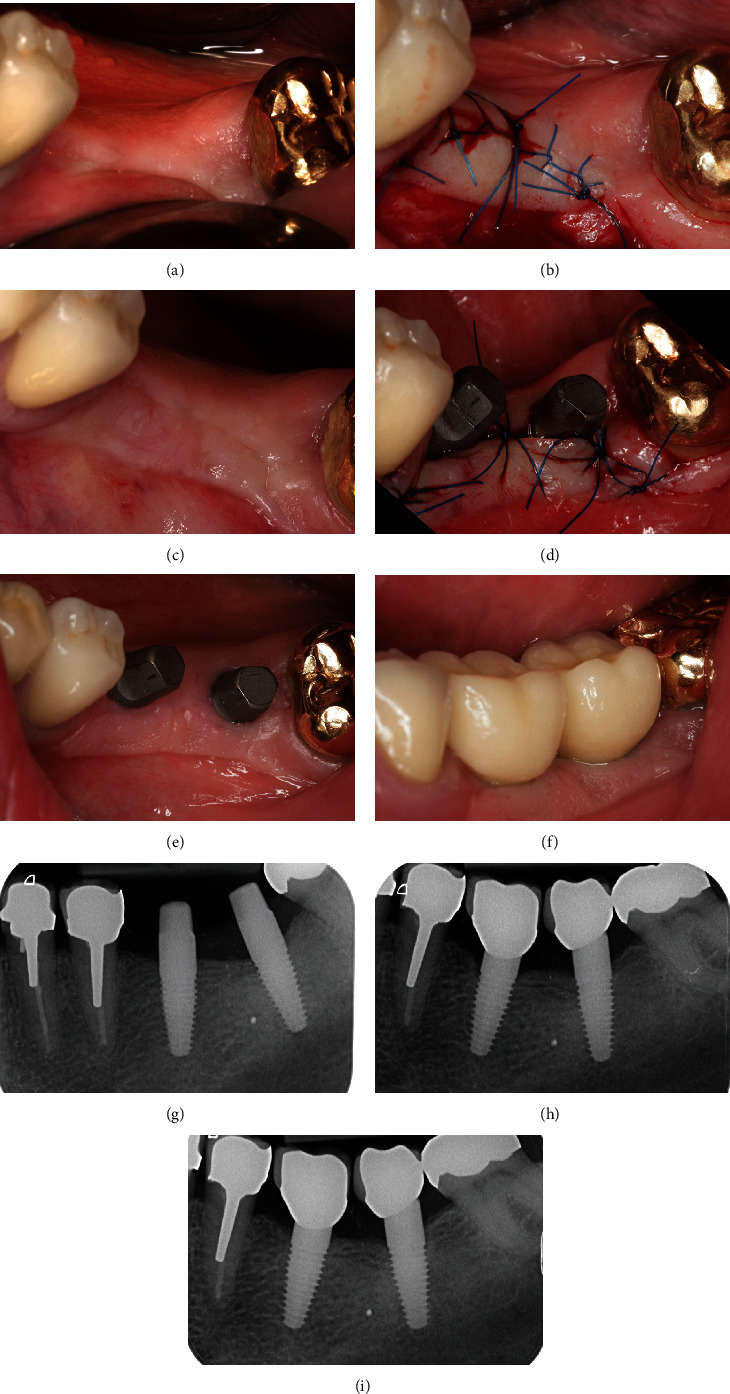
Soft-tissue augmentation in the region of the planned implantation: (a) clinical situation presurgically presenting small alveolar crest and reduced width of keratinized gingiva around implant site; (b) augmentation of free gingival graft at the buccal aspect; (c) clinical situation six weeks after healing; (d) postoperative clinical situation after implantation of two one-piece implants, flap closure; (e) clinical situation after 12 weeks of transgingival healing; (f) prosthetic restoration six months after implantation, cemented single crowns; (g) radiographic control after implantation; (h) postsurgical radiograph taken directly after cementation of single crowns; (i) radiographic control nine years after implant treatment.
